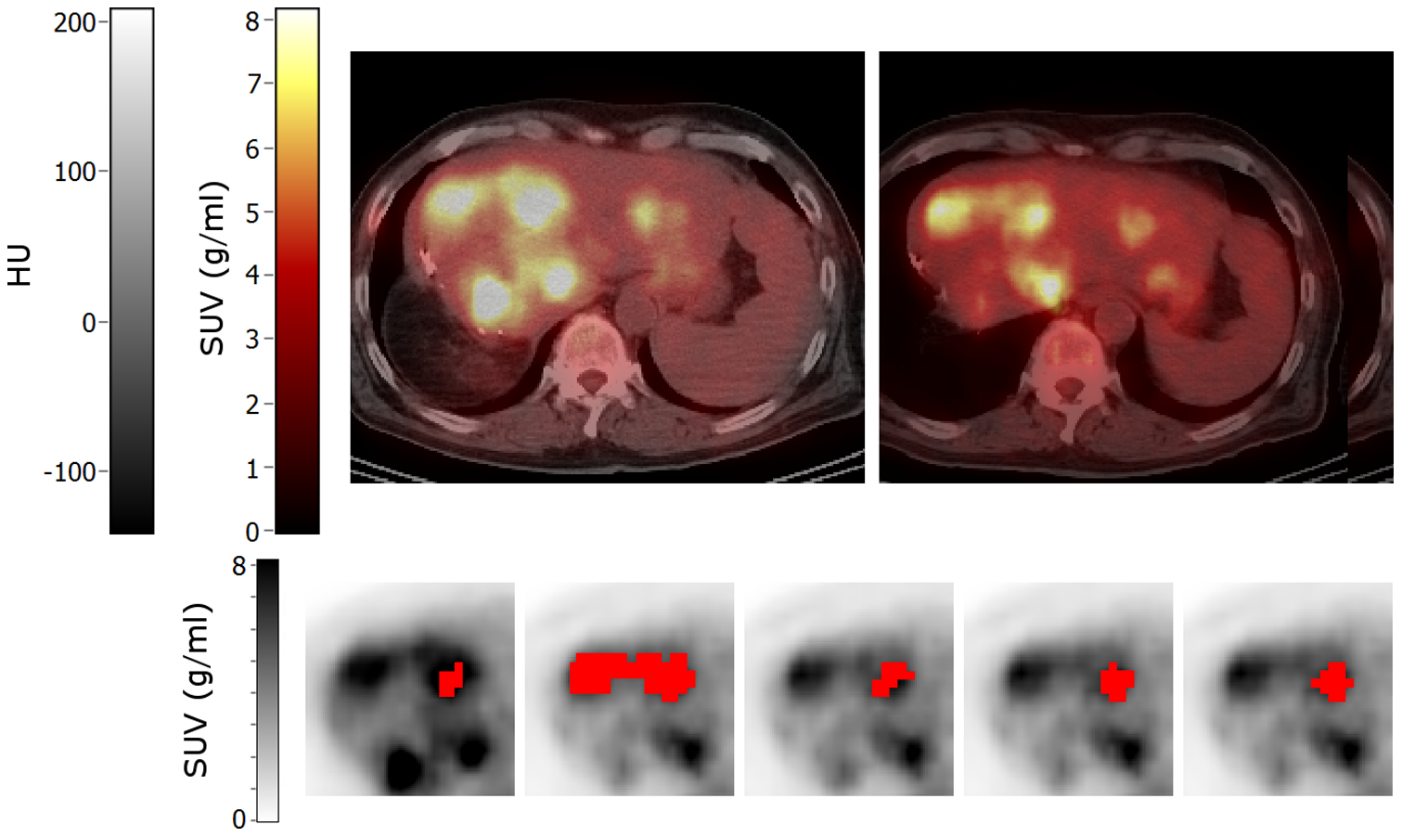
Axial images of a patient with liver metastases. Top row: baseline (left) and early response (right) PET/CT images. Bottom row: volumes of interest (shown in red) projected onto the baseline (first image) and early response scans (other images) that were obtained using (from left to right): A50 defined on baseline scan, A50 defined on early response scan, and local rigid PET, non-rigid PET and non-rigid CTPET image registration. All images are shown using the same colour scales. Abbreviations: SUV, standardized uptake values; HU, hounsfield units; A50, 3 dimensional (semi-)automatic isocontour method at 50% of the maximum pixel value that corrects for local background.
